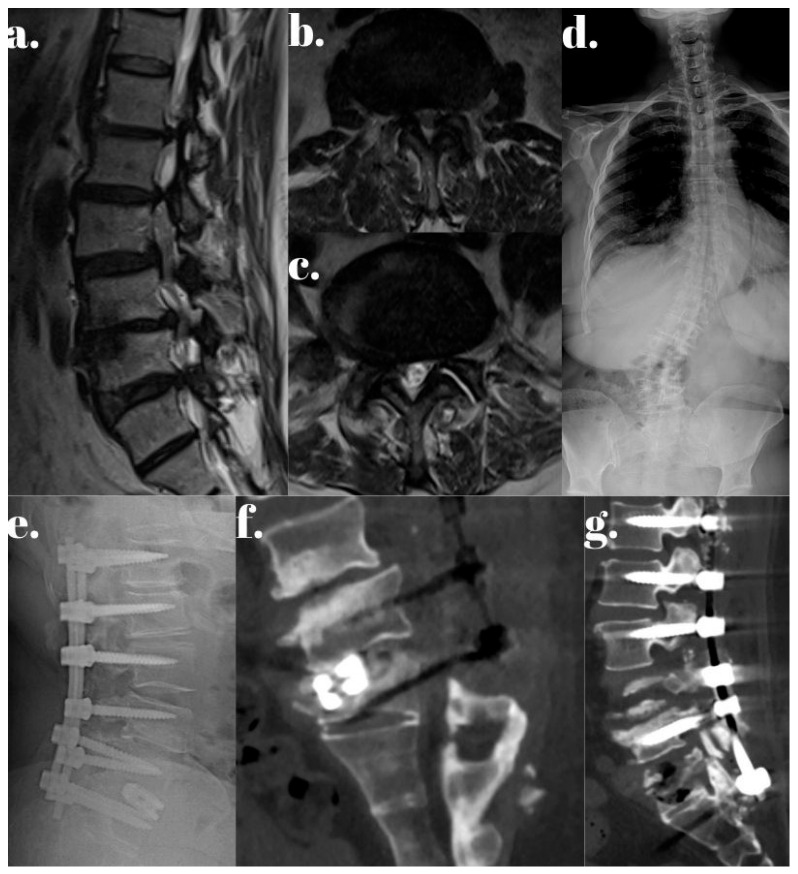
A patient who underwent L1–S1 rigid stabilization with TLIF for severe low back pain experienced worsening low-back pain at six months postoperatively. CT imaging revealed TLIF subsidence and S1 screw malposition. (a) Preoperative sagittal MRI image. (b,c) Preoperative axial MRI images. (d) Preoperative anteroposterior X-ray image. (e) Lateral X-ray image after the initial L1-S1 rigid stabilization with TLIF. (f) Sagittal CT image showing TLIF subsidence and S1 screw malposition. (g) Sagittal CT image following revision surgery (2nd year postoperatively).
